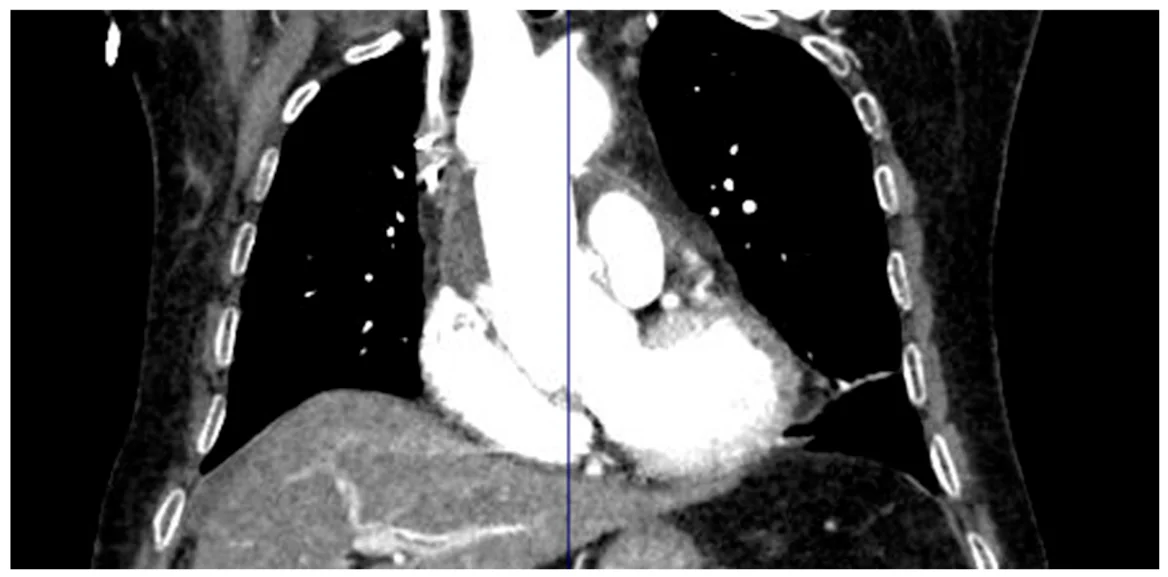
Postoperative contrast-enhanced chest CTA showing successful ascending aortic repair with the vascular graft and previously implanted TAVI prosthesis in situ. No contrast extravasation, residual dissection, anastomotic complications, or pericardial effusion are observed.
